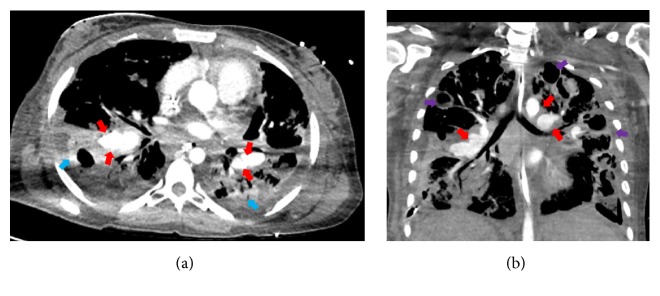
Computed tomogram angiography of the chest in (a) axial plane and (b) coronal plane depicting multiple saccular, contrast-enhanced structures close to the branches of the pulmonary arteries. These were found to be mycotic pulmonary artery pseudoaneurysms (red arrows). Bilateral, bibasilar dense consolidations from necrotizing* Staphylococcus aureus* pneumonia (blue arrows) and cavities secondary to necrotizing* S. aureus* pneumonia (purple arrows) are also seen.
